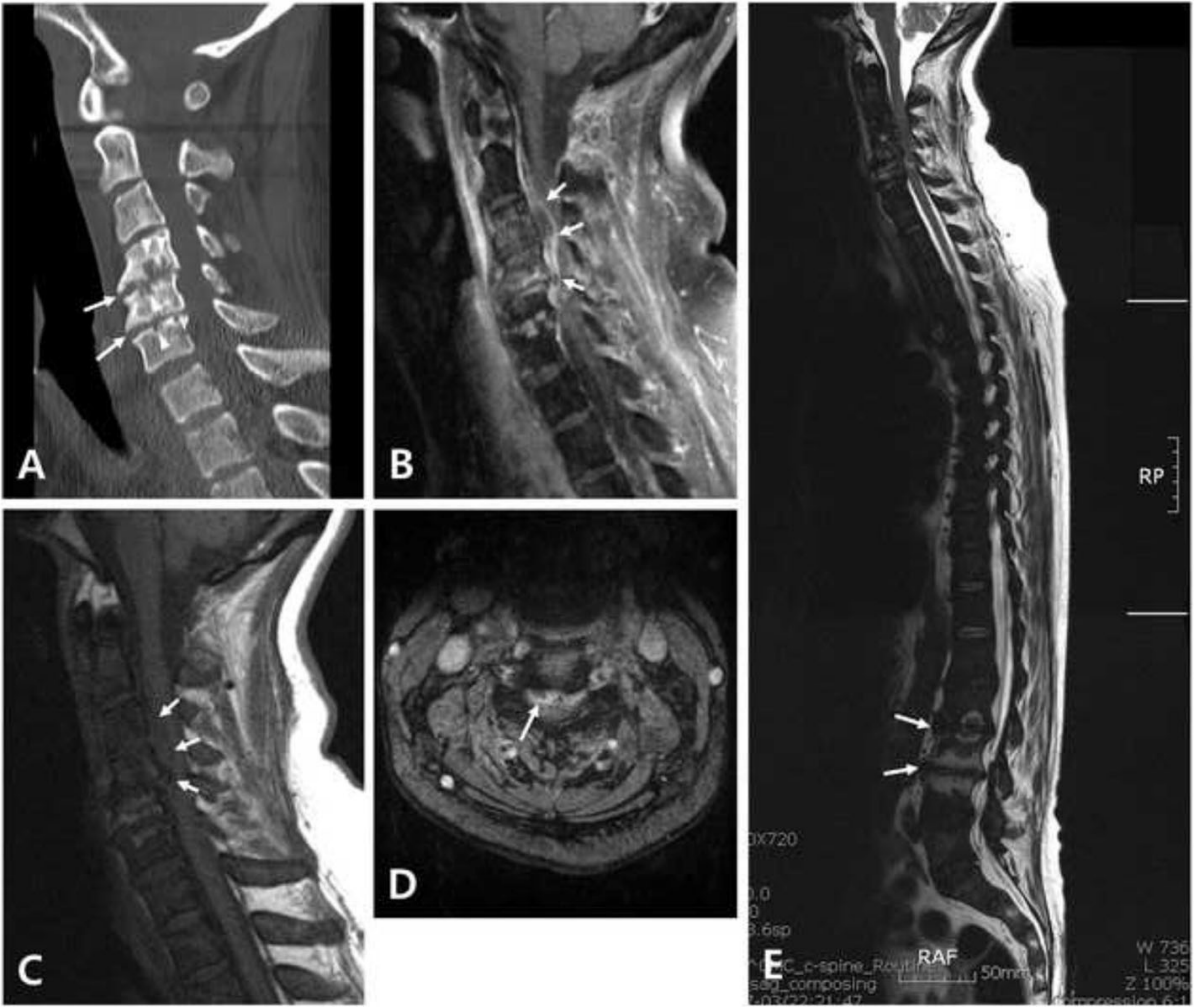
Preoperative images. a Sagittal reconstructed computed tomography scan of the cervical spine demonstrates the typical findings of destructive spondyloarthropathy, such as intervertebral disc space narrowing, osteophytes (*arrows*), and erosion and cysts (*arrow heads*) in the adjacent vertebral endplates at the level of C4-C6. b Gadolinium enhanced T1-weighted sagittal, c T1-weighted sagittal, and d T2-weighted axial magnetic resonance (MR) images reveal the epidural abscess (*arrows*) causing spinal cord compression at the C3-C5 level. e T2-weighted whole-spine sagittal MR image also demonstrates the typical findings of destructive spondyloarthropathy, such as intervertebral disc space narrowing, minimal osteophyte formation, and erosion and cysts in the adjacent vertebral endplates at the L2-L4 level (*arrows*)
